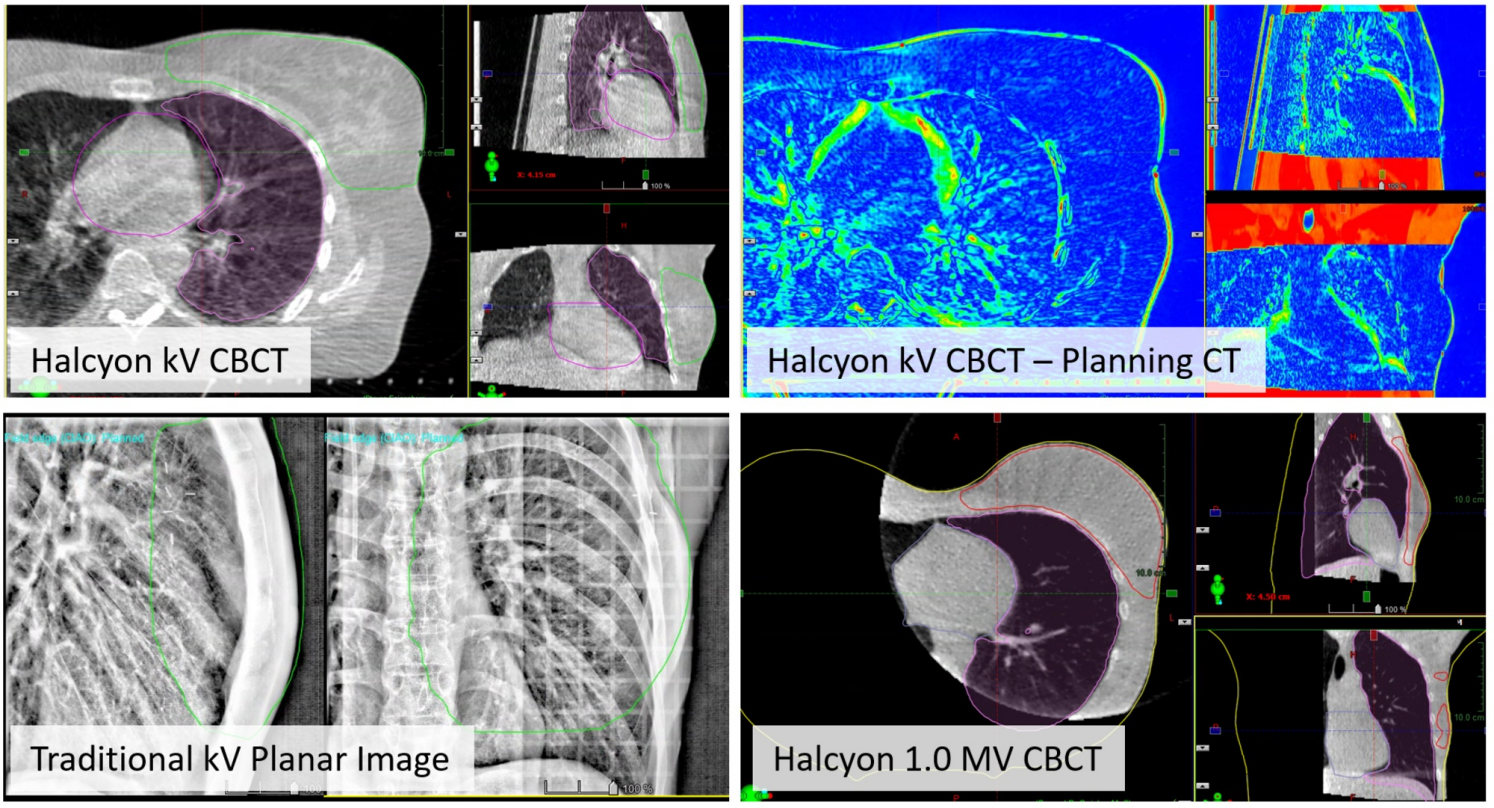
Top row: Online IGRT with improved kV CBCT of the tangent fields using the breast CBCT preset. Overlays of the heart, ipsilateral lung, and BREAST_PTV structures from the planning CT allow assessment of alignment quality. The image on the right shows the difference between planning CT and online CBCT. Bottom row: Examples of breast localization with traditional kV planar image pair and Halcyon 1.0 MV CBCT IGRT: image-guided radiation therapy; CBCT: cone beam computed tomography; PTV: planning target volume
